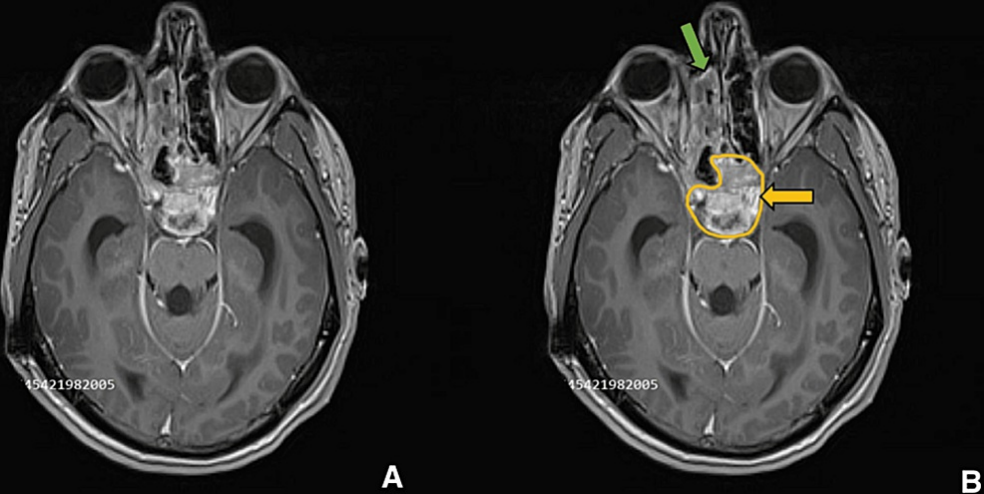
Preoperative imaging for patient 1. Contrast-enhanced T1-weighted axial image showing sellar, suprasellar lesion centered over clivus, measuring 4.5 x 5.1 x 3.1 cm. (A) Without ROI, and (B) with ROI. The yellow arrow points to the lesion which extends anteriorly to invade the sphenoid sinus. The green arrow shows the involvement of the right ethmoid sinus.
